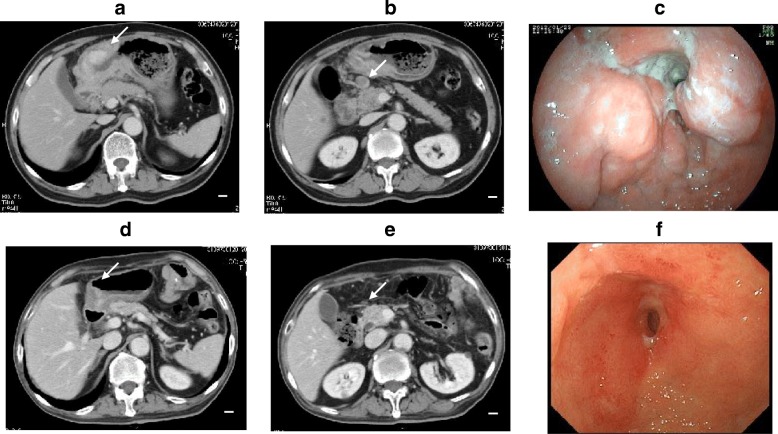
Computed tomography (CT) and esophagogastric fiber (EGF) findings at diagnosis and after two courses of docetaxel, cisplatin, and TS-1 chemotherapy. a, b Abdominal CT showed thickened wall of the antrum and a bulky lymph node (*arrows*). c EGF showed type 3 tumor in the antrum. d, e Thickened antrum wall was significantly improved, and the bulky lymph node was significantly shrunk (*arrows*). f Type 3 tumor was remarkably shrunk. Side bar = 1 cm
